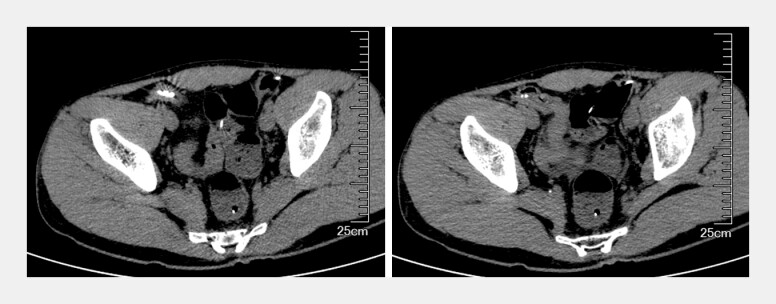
CT showed no free gas or exudation in the terminal ileum 4 days after perforation. CT, computed tomography.
